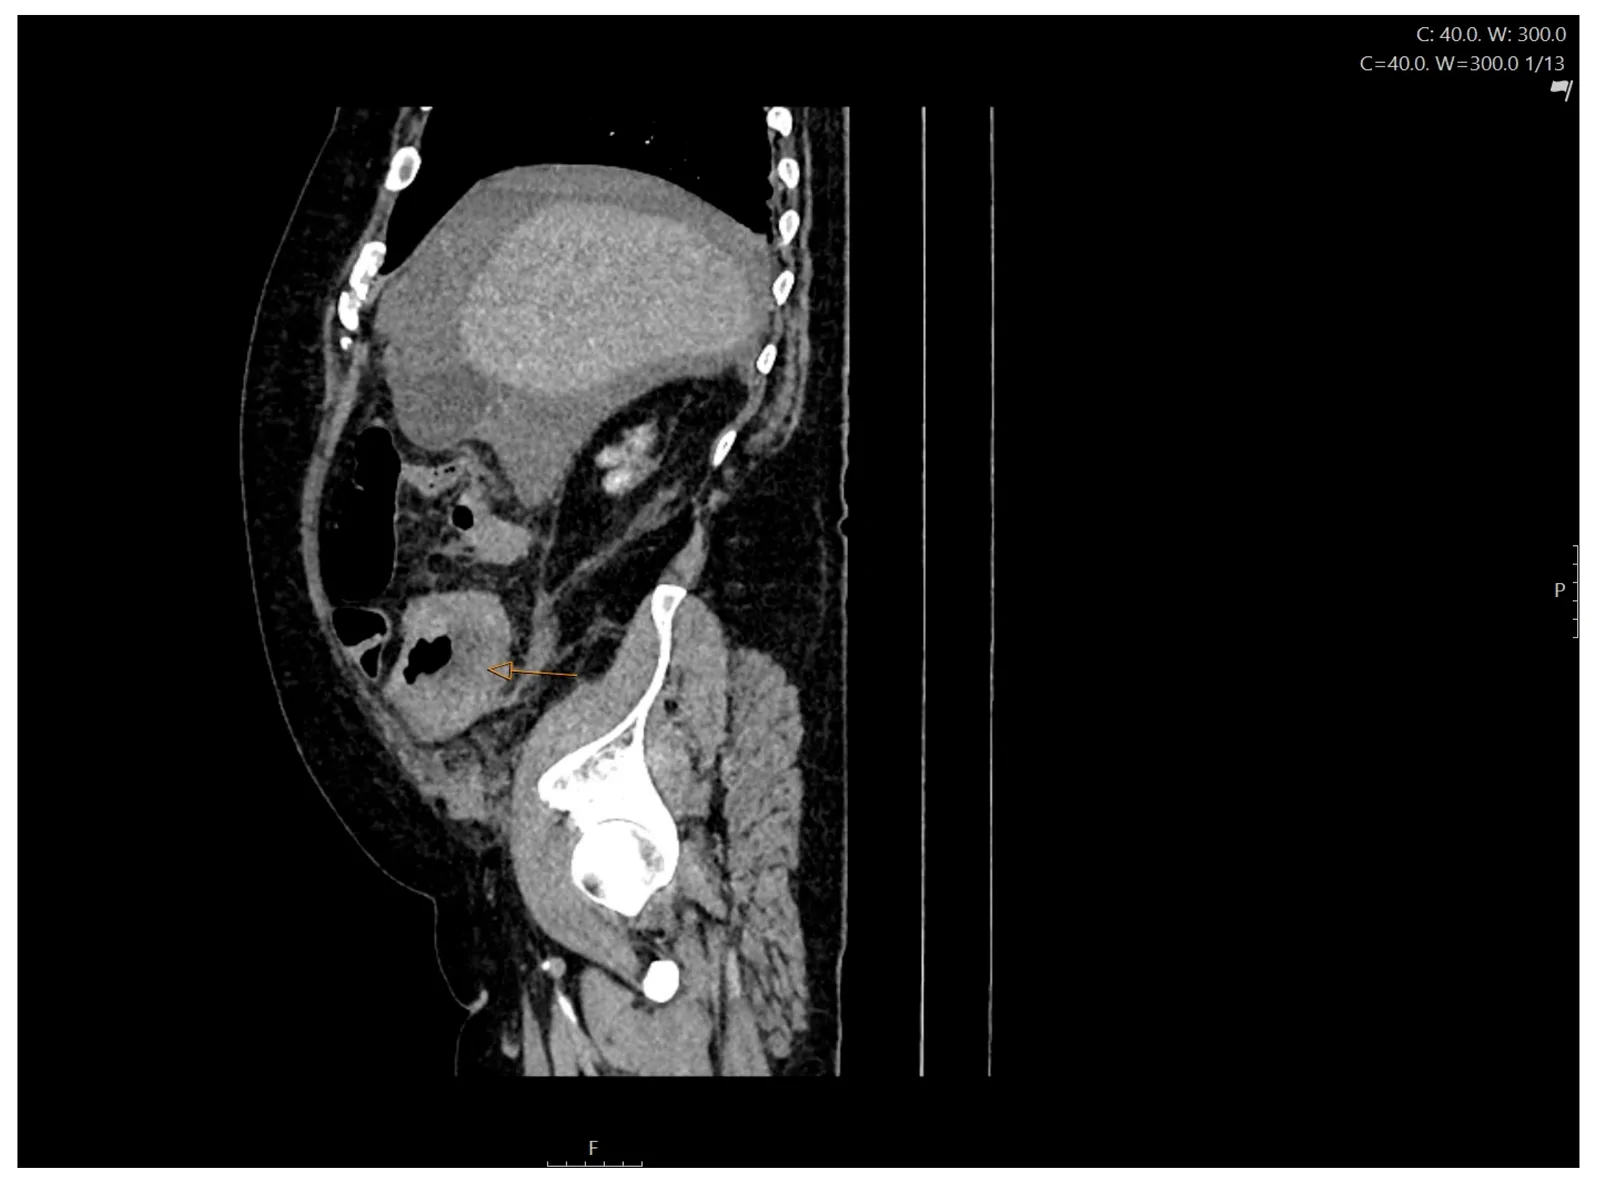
CT scan showing ascites and eccentric wall thickening of the cecum and terminal ileum with a large hypovascular area consistent with a necrotic tumor extending approximately 6 cm craniocaudally (yellow arrow).
